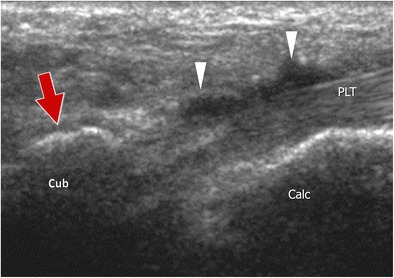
Normal os peroneum – ultrasound. The transducer is moved forward along the PLT until the cuboid (*cub*). A small amount of synovial fluid can be visualized along the PLT (*arrowhead*). When present, the OP is visualized at this level as a hyperechoic structure showing posterior shadowing (*arrow*)
